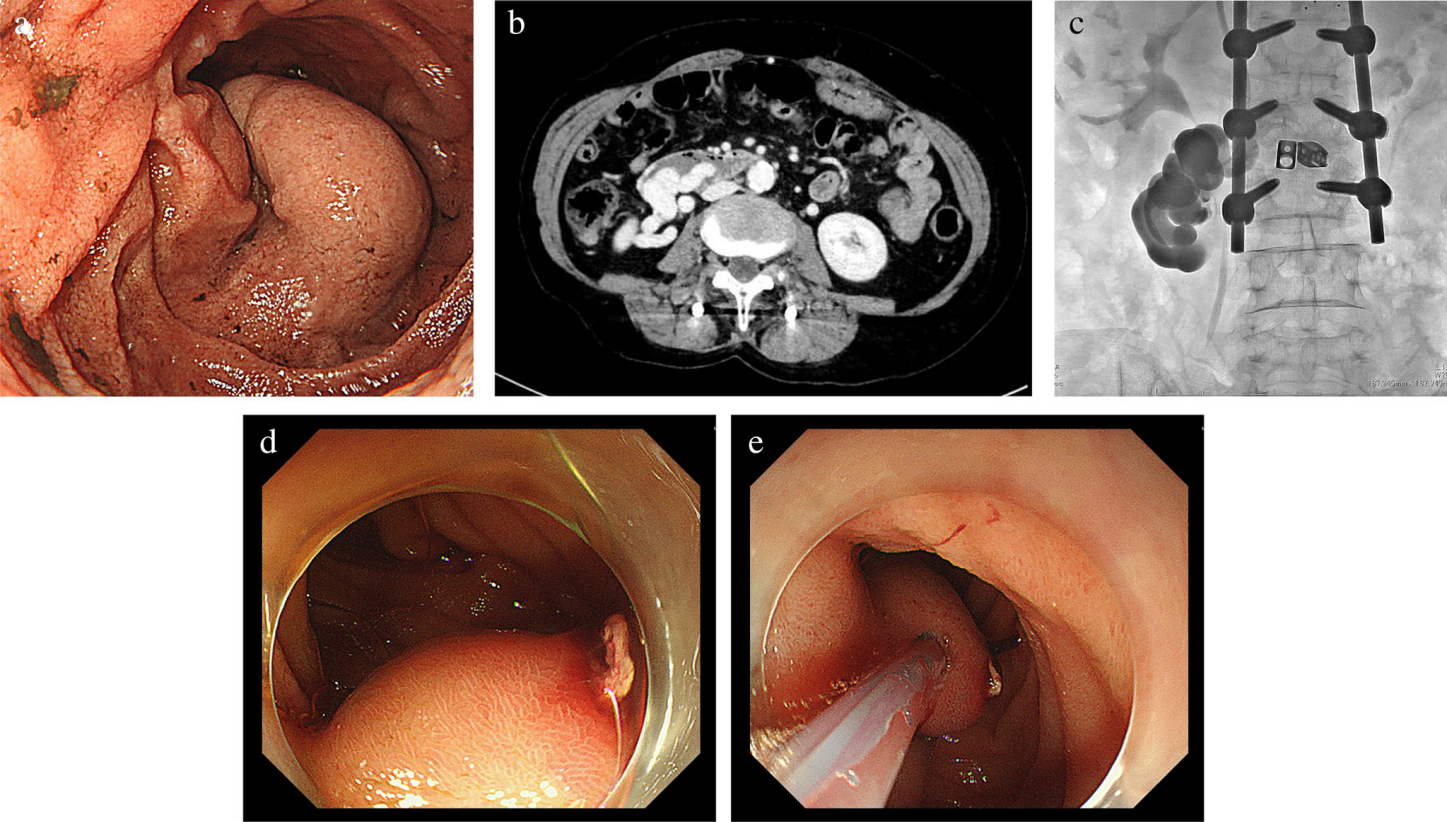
(a) Duodenal varices on admission to a local hospital. (b) Contrast‐enhanced computed tomography (CT) revealed that varices were located in the second portion of the duodenal wall including dilated superior mesenteric veins as a feeding vein and dilated ovarian veins as a drainage vein. (c) B‐RTO showed that good pooling of ethanolamine oleate with iopamidol was observed in duodenal varices using balloon catheters. (d) Emergent gastroduodenoscopy revealed a white plug on the treated varices. (e) A total of 1.5 mL of 66.6% cyanoacrylate was injected carefully into the duodenal varices, and hemostasis was completely achieved.
